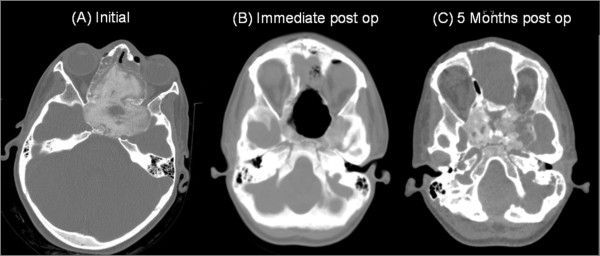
Series CT scans of the tumor. (A) preoperative CT revealing ground glass appearance is consistent with fibrous dysplasia affecting of the sphenoid bone, ethmoidial bones, LT maxillary sinus, involving the optic foramen and harboring cystic expansible component. (B) Immediate post operative CT scan. (C) 5 months post operative follow up CT scan.
